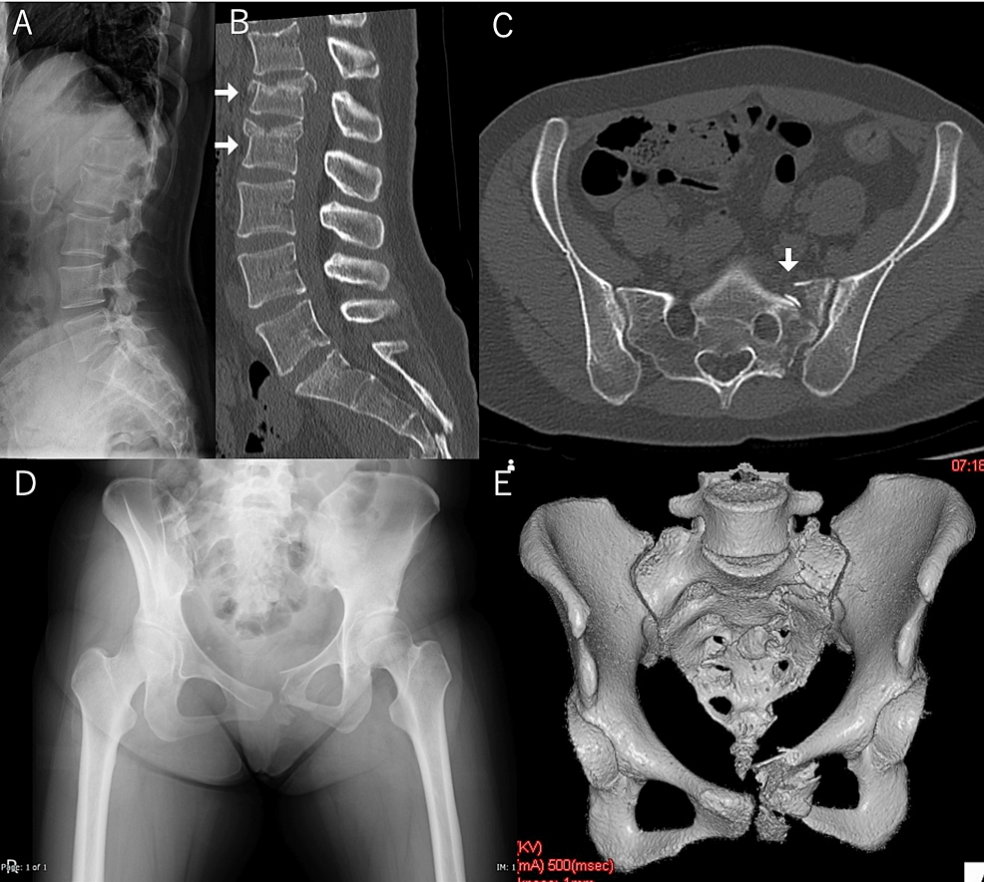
Findings at the time of transport to our hospital. (A) Lateral X-ray view shows fracture of the L1–2 vertebrae. (B) CT sagittal view of the spine shows the burst fracture of the L1–2 vertebrae and T12 spinous process (AO type classification; type B2) (white arrows). (C) CT axial view of the pelvis shows a left sacral fracture (white arrow). (D) Frontal X-ray shows a pelvic ring fracture. (E) Three-dimensional CT reconstruction shows a lateral compression type 1 pelvic ring fracture. CT, computed tomography
